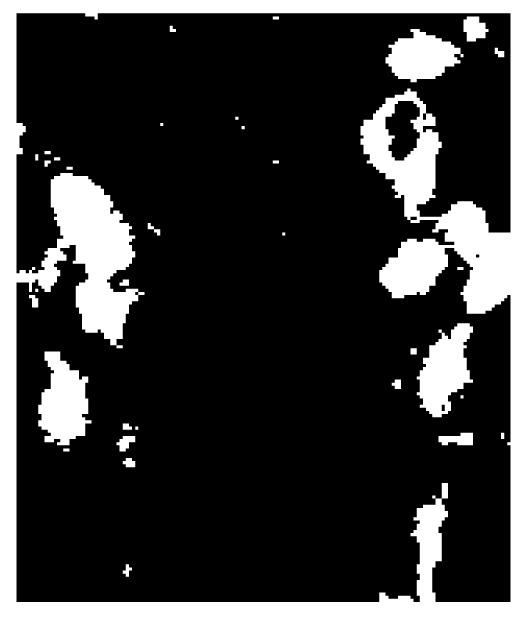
Binary segmentation of the brain MRI using gray-level threshold of 0.4.
